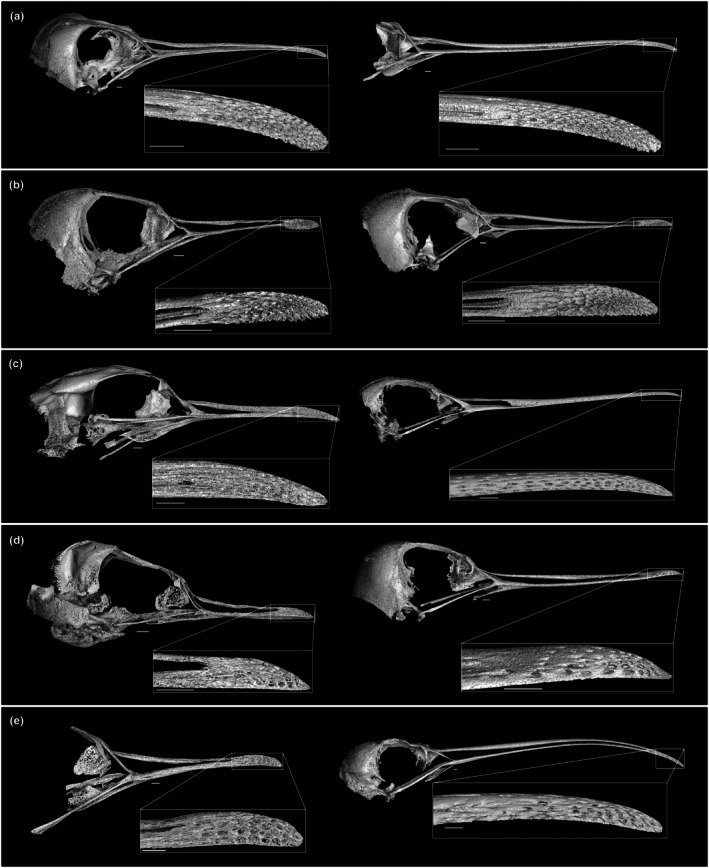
The skulls of five species of scolopacid shorebirds in lateral view taken from CT scans (juveniles on left, adults on right), with insets showing the tip of the bill to highlight the numerous neurovascular pits in the bone. (a) *Calidris ferruginea*; (b) *C. minuta*; (c) *Tringa erythropus*; (d) *Phalaropus fulicarius*; (e) *Numenius phaeopus*. Scale bars = 1 mm.
